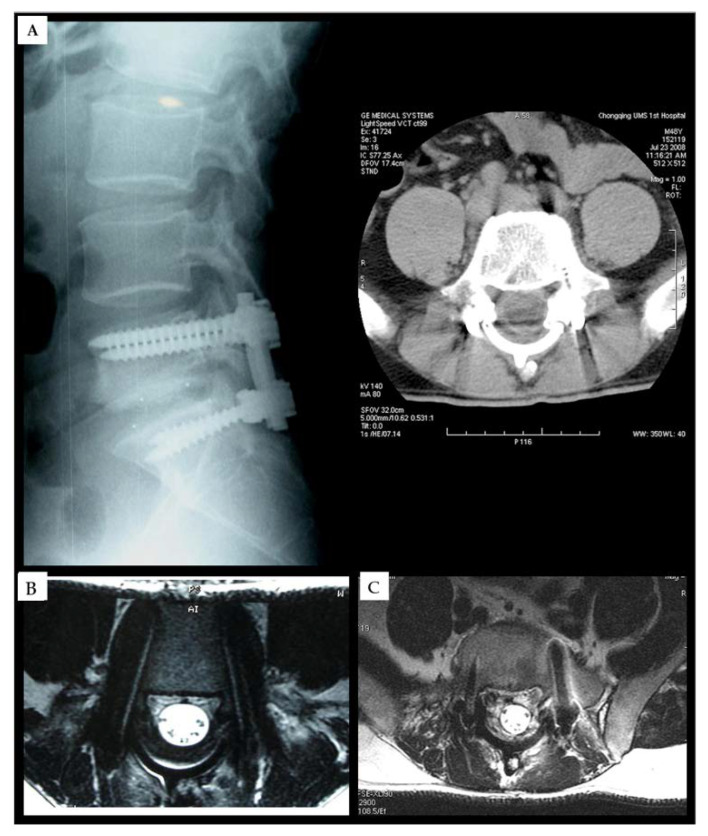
(A) The postoperative X-ray films showed that the internal fixation was in a good position with a good spinal alignment. (B) The postoperative CT and MRI of the post-op showed that the lumbar vertebral canal had good morphology and was covered by the n-HA/PA66 artificial lamina. (C) The postoperative axial MRI of the post-op and follow-up at 5 years showed no epidural scar formation and no compression of the nerve root. Reproduced with permission from Medical Science Monitor, Copyright 2018 [75].
